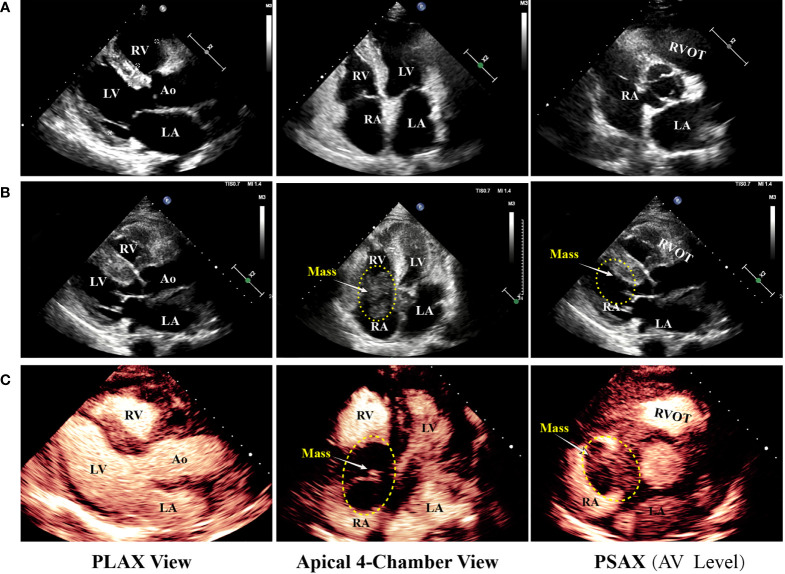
Echocardiography progression of the patient. (A) The patient underwent echocardiography 2 months prior to the present hospitalization, and no remarkable abnormality was revealed. (B, C) Regular TTE and myocardial contrast echocardiography showing tumor extending from the right atrium to the right ventricle through the tricuspid valve. LA, left atrium; LV, left ventricle; RA, right atrium; RV, right ventricle; Ao, aorta; RVOT, right ventricular outflow tract; PLAX, parasternal long axis; PSAX, parasternal short axis; AV, aortic valve.
